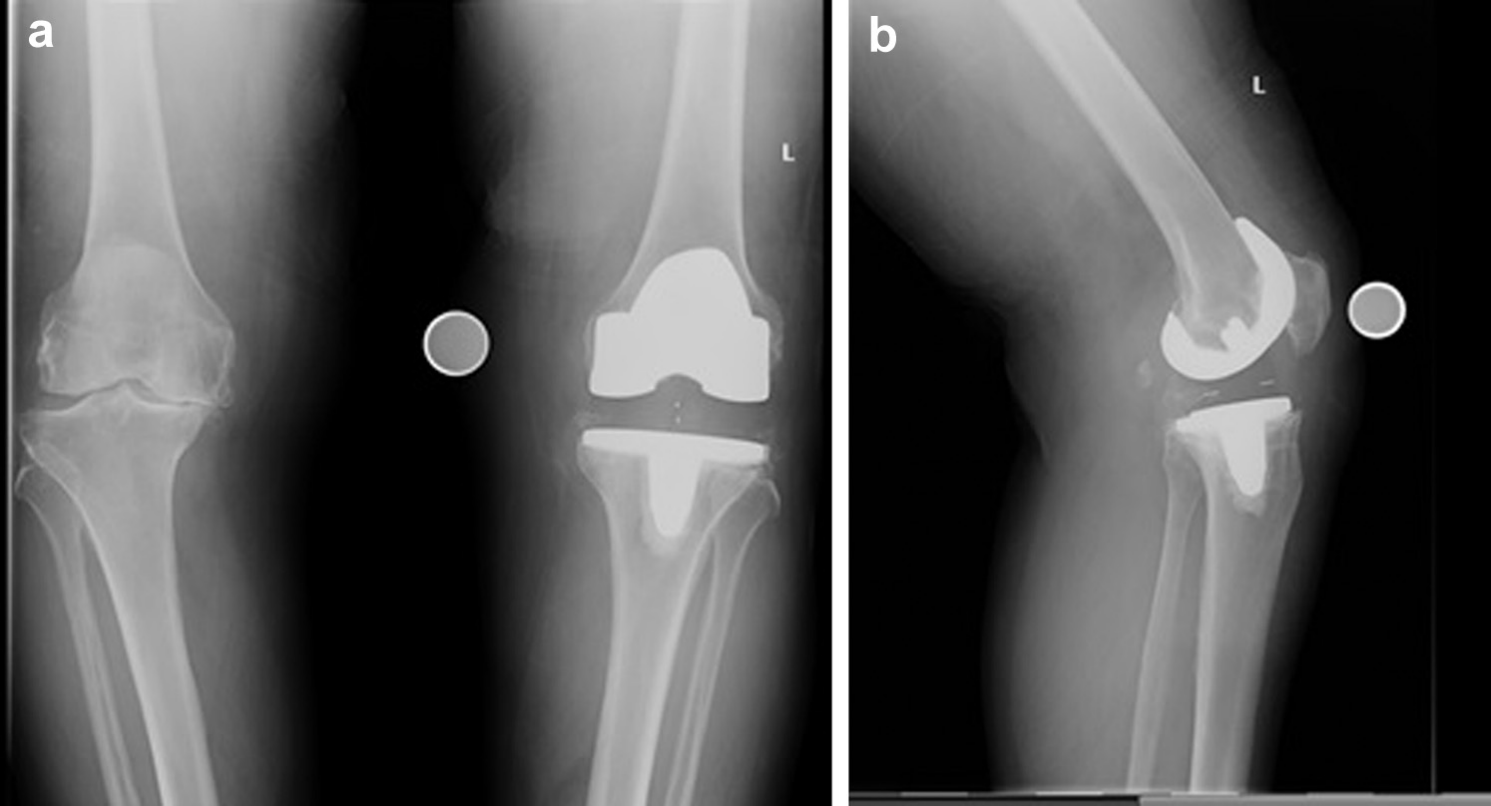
(a) Anteroposterior and (b) lateral radiographs of left TKA at presentation 10 years after index surgery showing loosening on the lateral aspect of the tibial tray.
